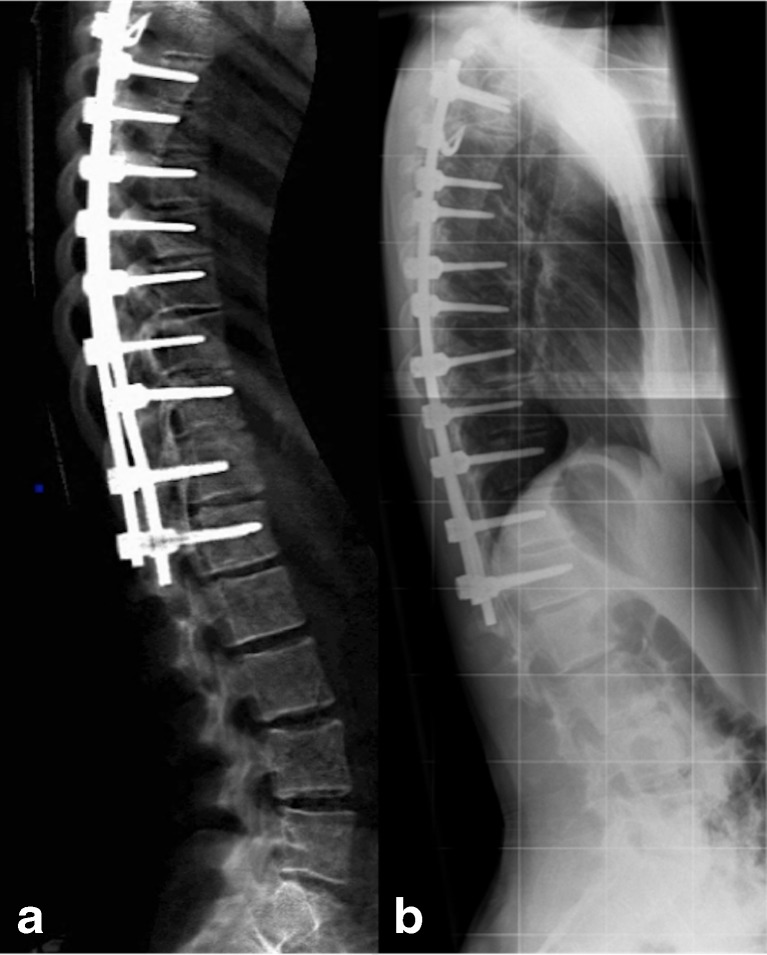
Lateral iDXA (a) and thoracic spine radiograph (b) of patient 80, a 14-year-old female with adolescent idiopathic scoliosis and previous spinal fixation. All observers independently scored vertebrae T4 to T6 as not fractured on iDXA. All observers were independently unable to score T4 to T6 on radiography because of poor image quality
